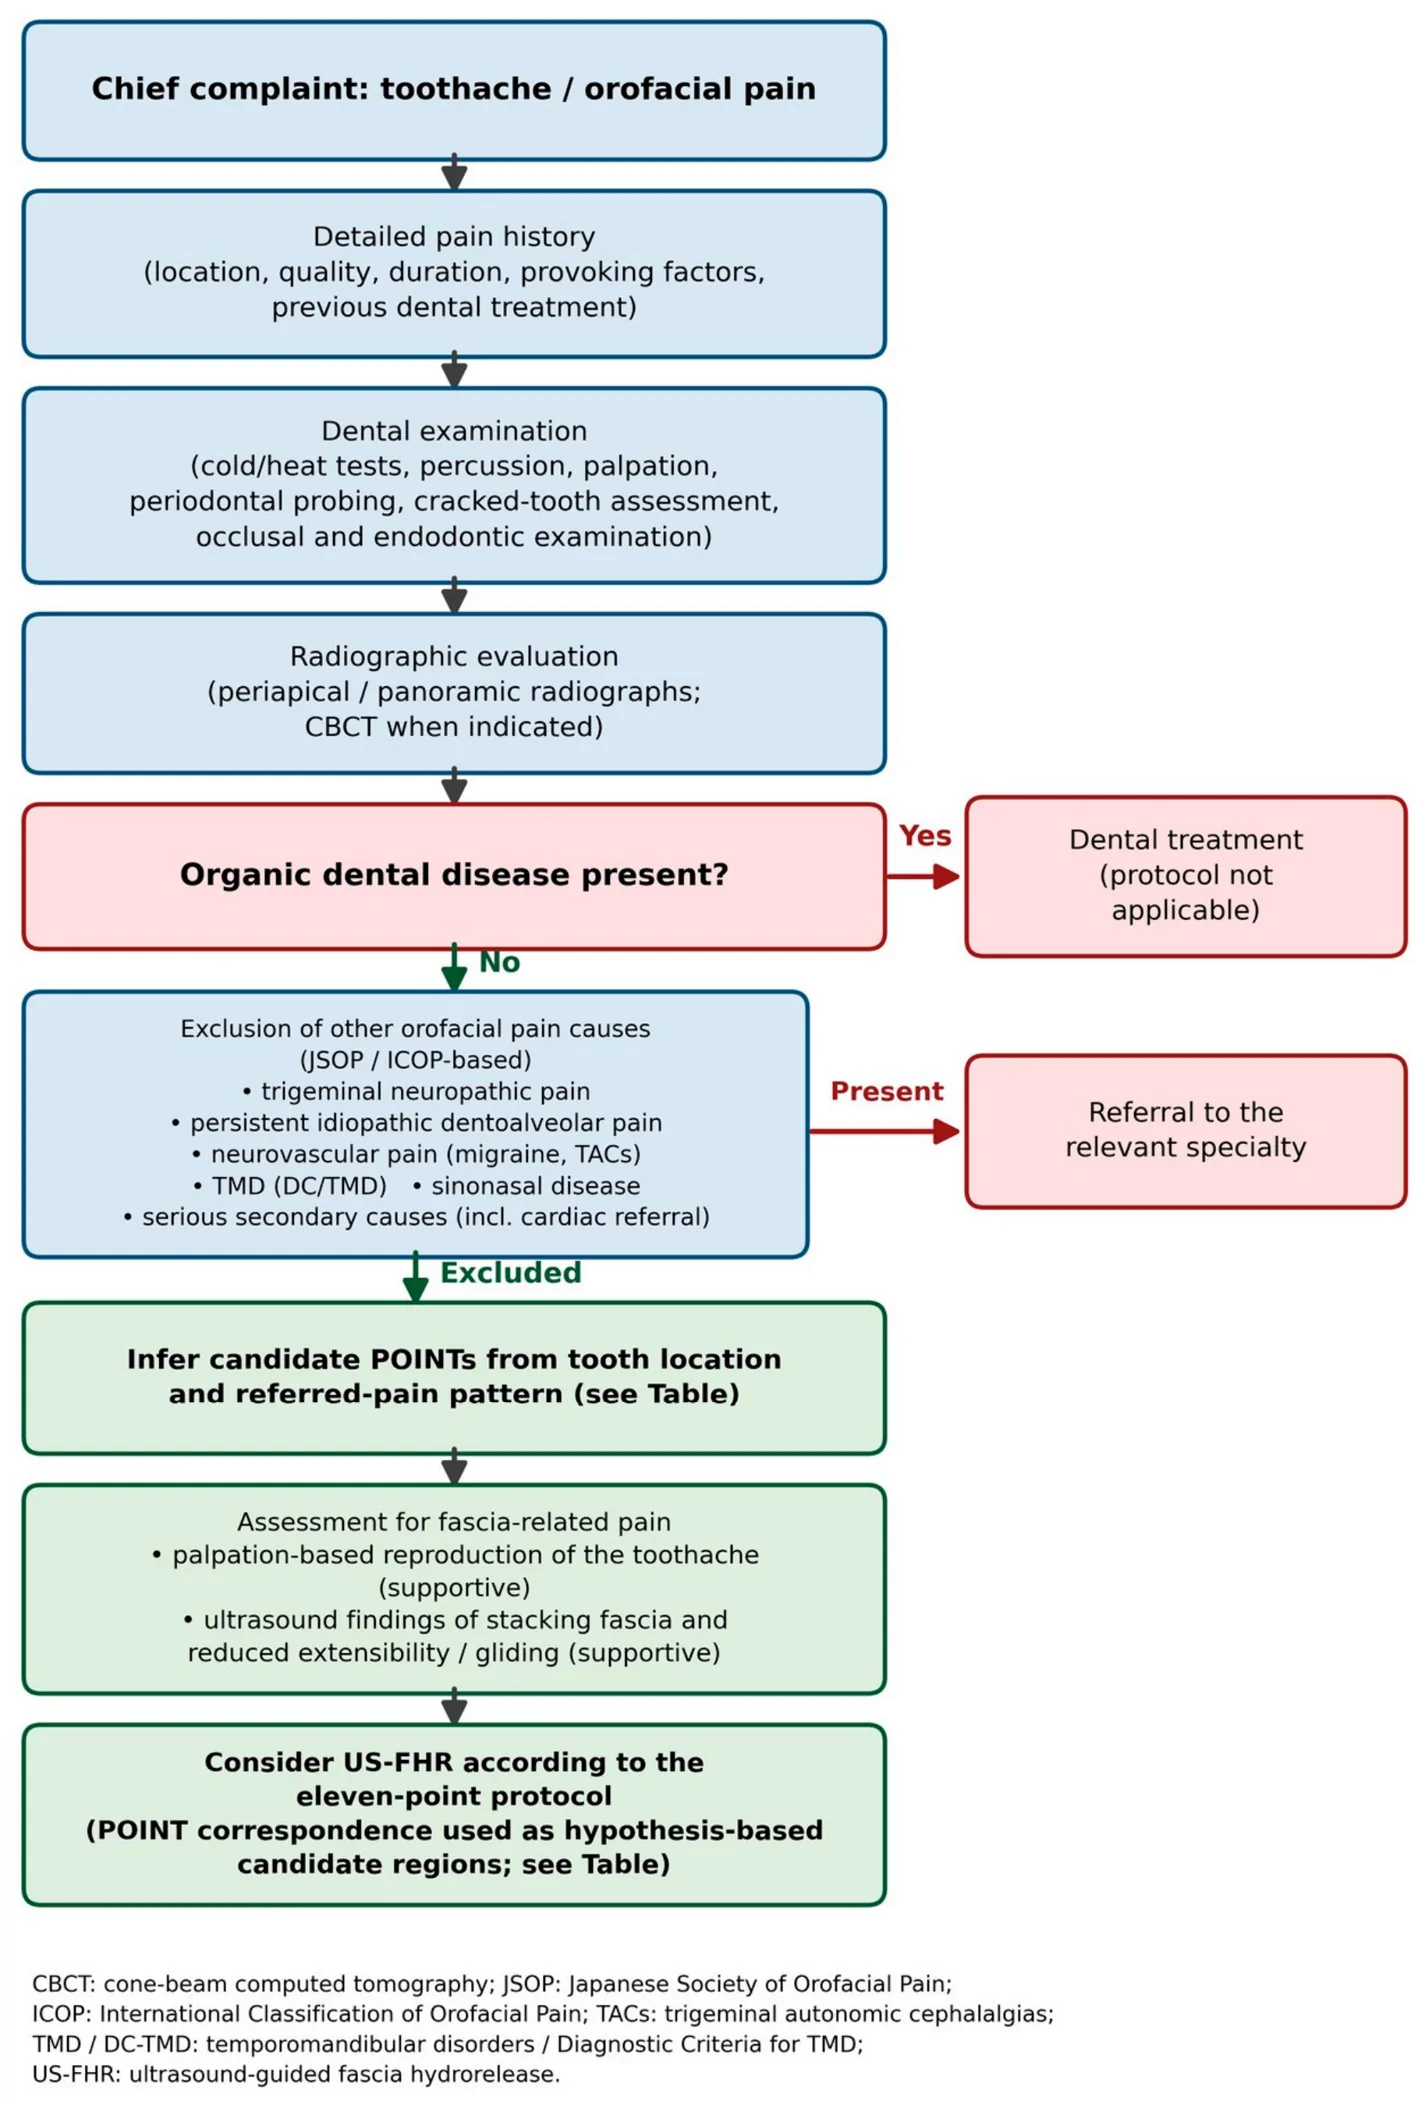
Diagnostic algorithm for non-odontogenic toothache: stepwise exclusion of odontogenic and non-fascial causes before consideration of ultrasound-guided fascia hydrorelease. The algorithm incorporates the International Classification of Orofacial Pain (ICOP) [13] and the Diagnostic Criteria for Temporomandibular Disorders (DC/TMD) [14]. Neither the palpation-based reproduction of the toothache nor the ultrasound findings of stacking fascia is confirmatory; sensitivity and specificity have not been established.
